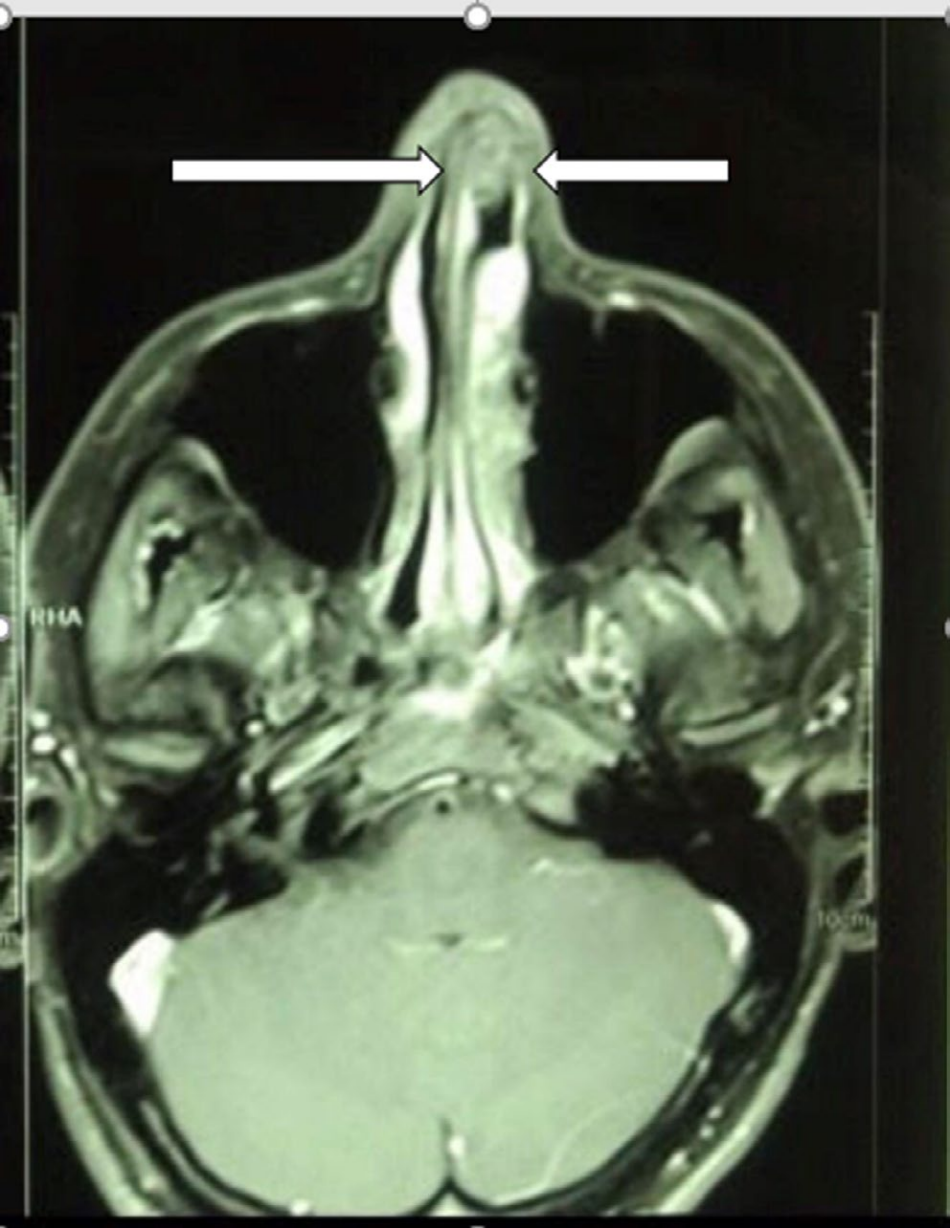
IV contrast T1‐weighted MRI. nonenhancing mass in left anterior part of the nasal septum
